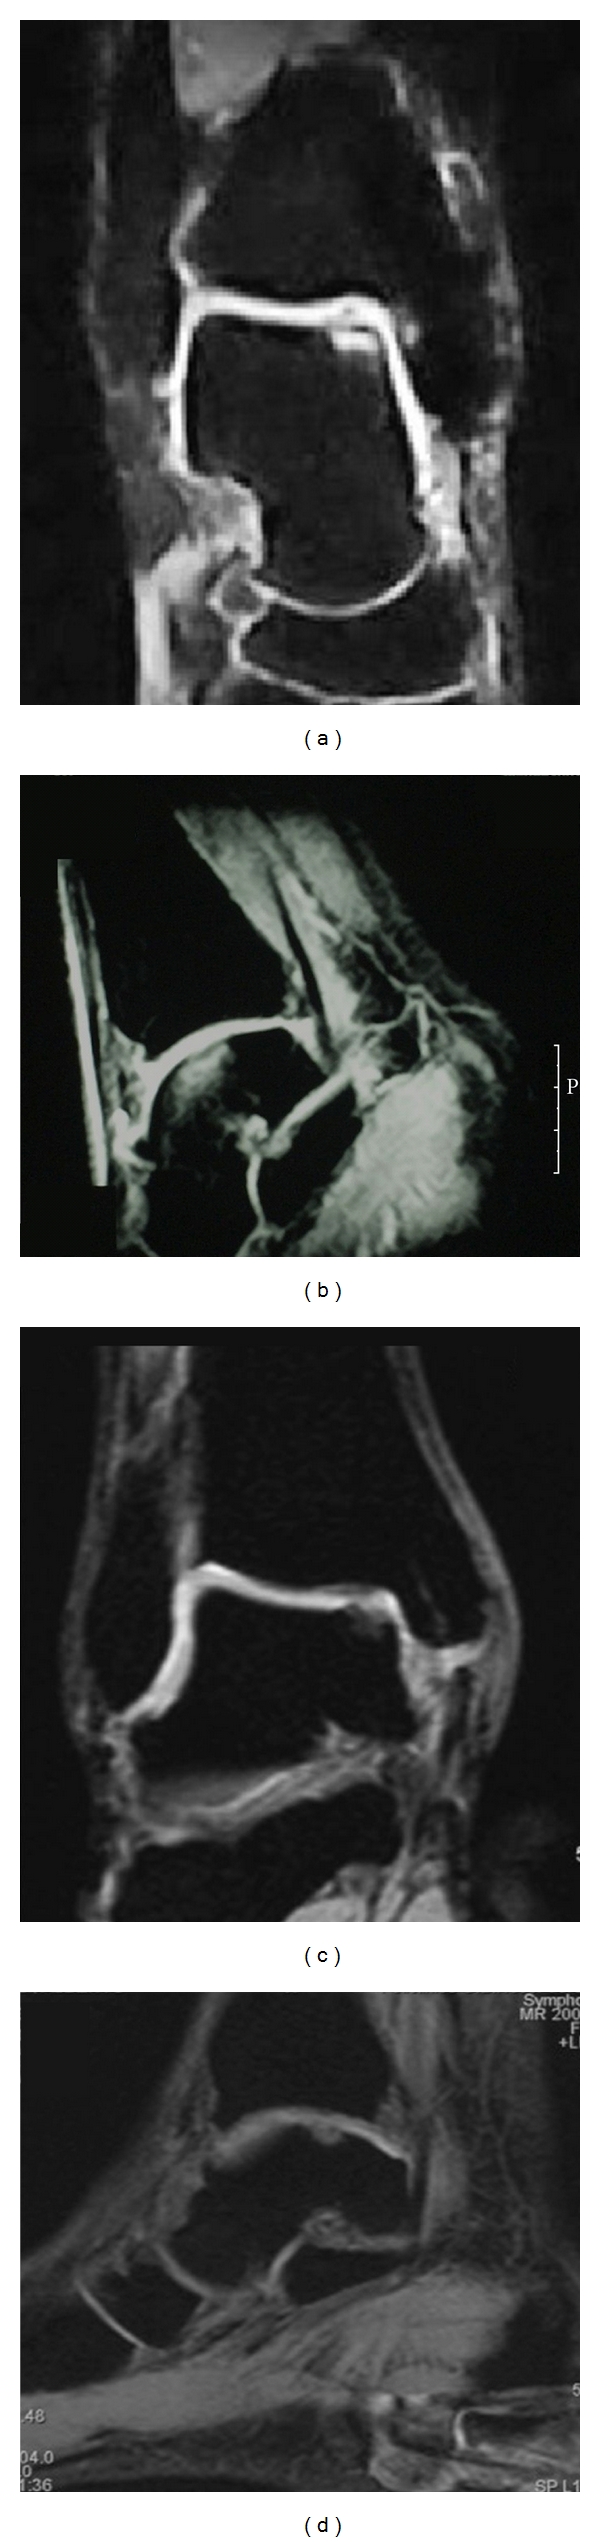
(a, b, c, d) Clinical case: 38-years-old man with anteromedial osteochondral lesion of the talus. Preoperative MRI images (a, b). AOFAS score was 30 points. (c, d) MRI images at follow-up (36 months). AOFAS score was 90 points.
